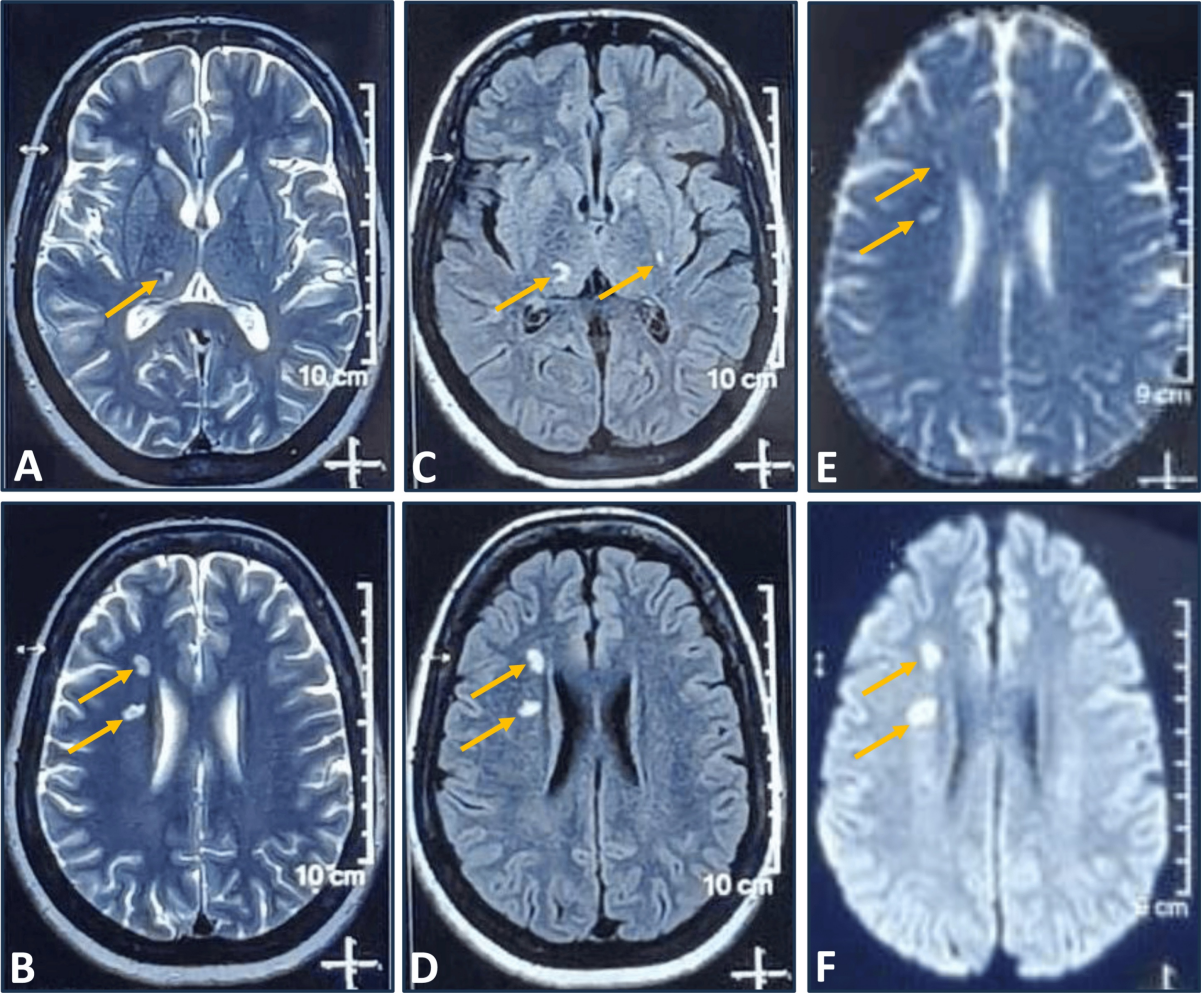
Axial MRI brain T2 (A, B) and FLAIR (C, D) images show a few foci of T2/FLAIR hyperintensities in the right frontal periventricular white matter, right thalamus, and left basal ganglia without restricted diffusion suggestive of old lacunar infarcts. The frontal lesions appear DWI hyperintense (E) without corresponding ADC hypointensity (F), suggestive of subacute–chronic infarcts. MRI: magnetic resonance imaging; T2: T2-weighted; FLAIR: fluid-attenuated inversion recovery; DWI: diffusion-weighted imaging; ADC: apparent diffusion coefficient.
